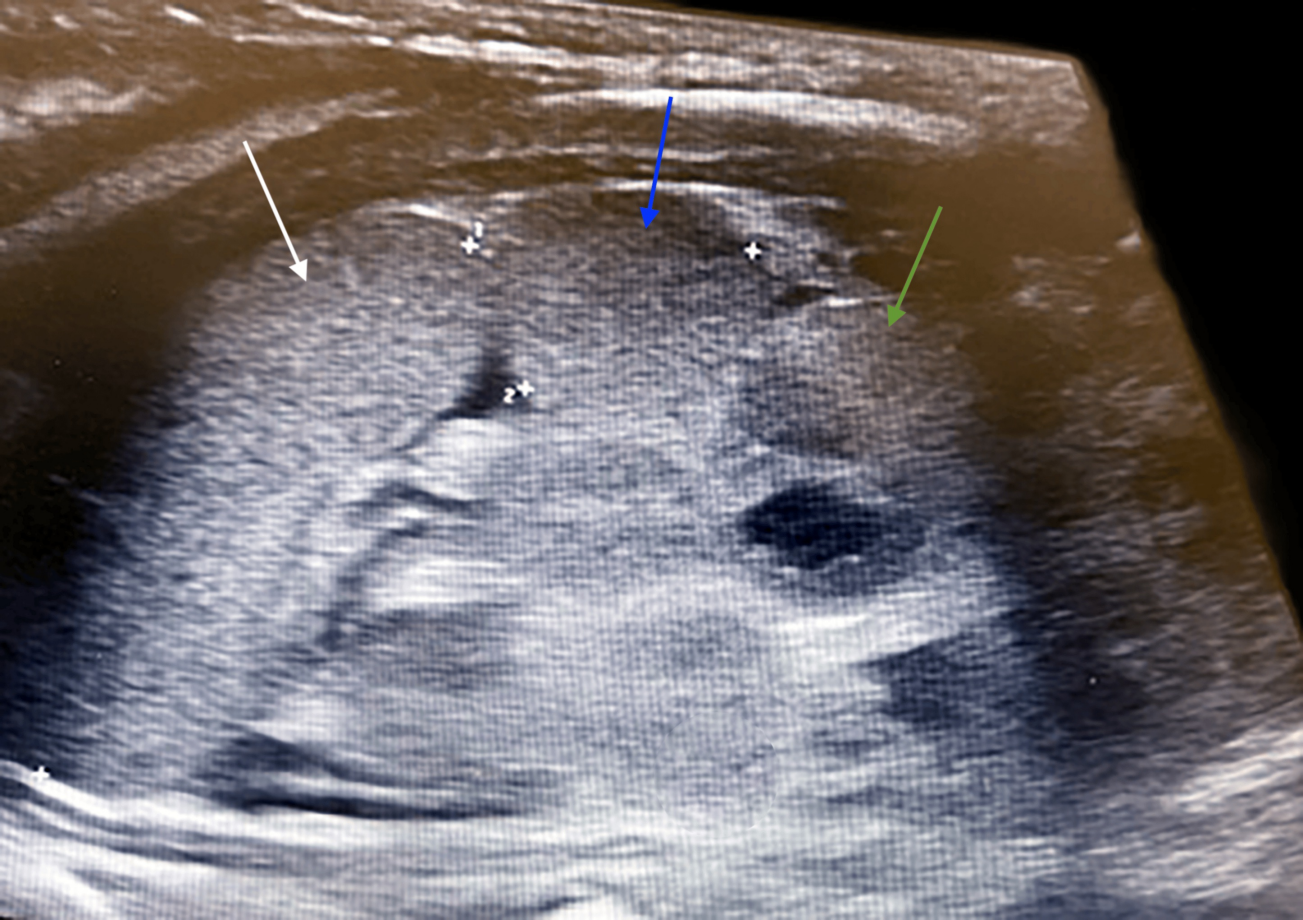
Longitudinal ultrasound view demonstrating polysplenia (white, blue, and green arrows)
